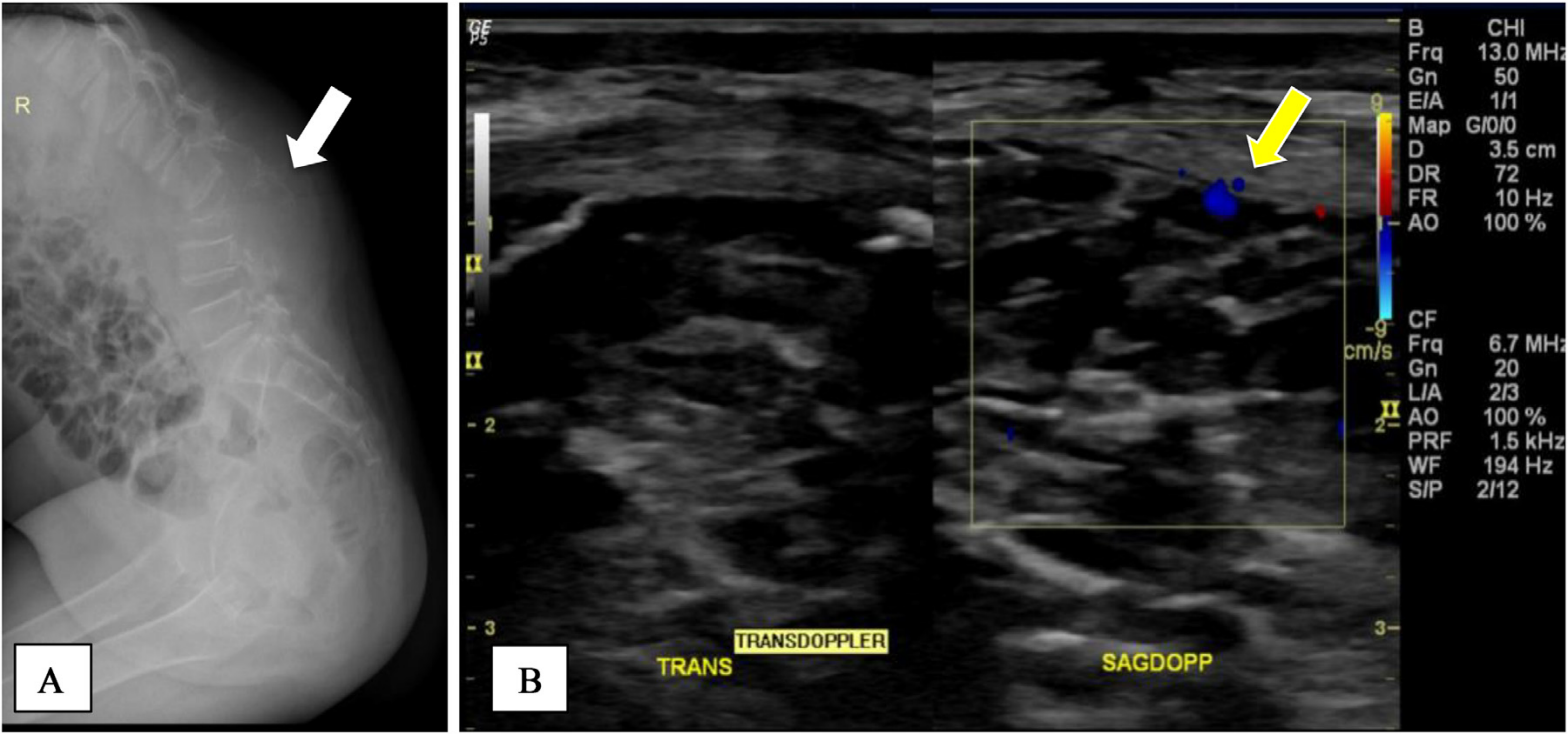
The x-ray (A) before the surgery showed a soft tissue mass without calcification extending from lumbar vertebrae 3-5 in the posterior lumbar region (white arrow). Ultrasonography with Doppler color (B) showed intramass vascularity (yellow arrow).
